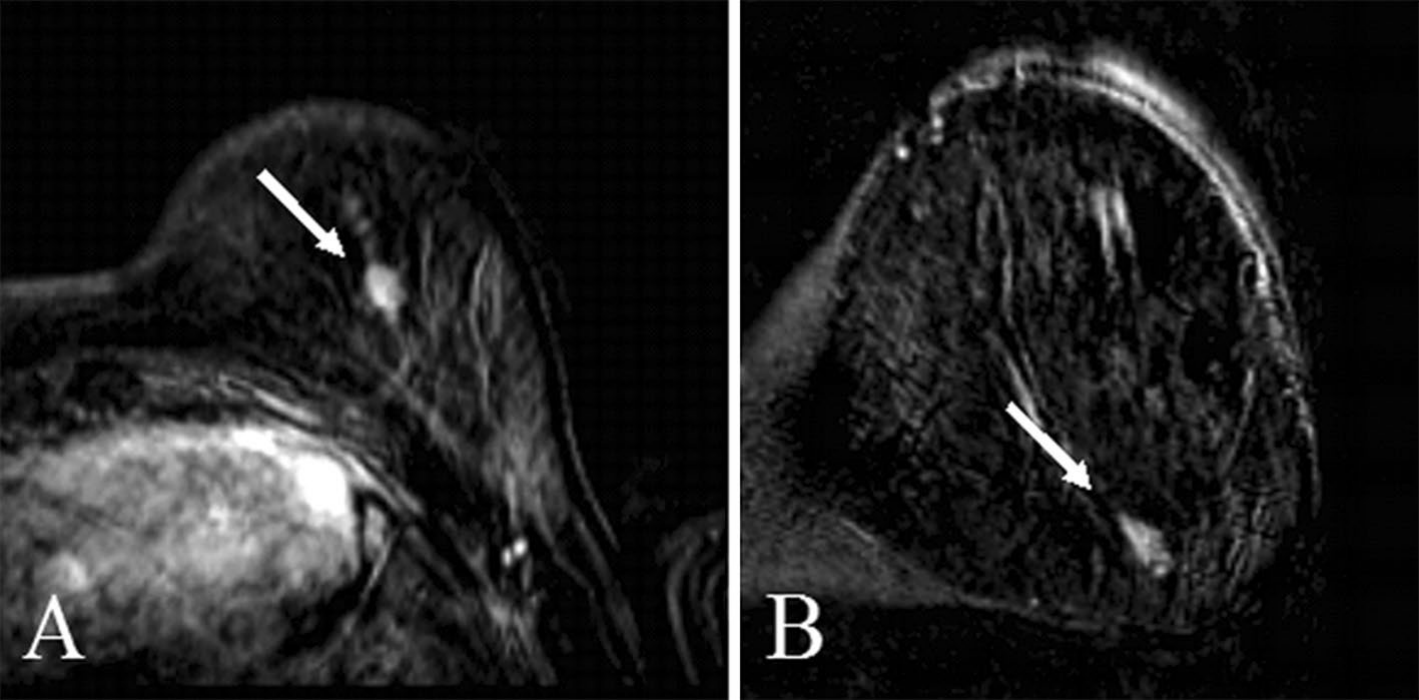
An example of an interval cancer detected on ultrasound 9 months after prior MRI. This visible lesions was scored as BI-RADS 5 in re-evaluation; a subtraction image of an invasive ductal carcinoma grade 2 (pT1cN1mi (sn)) in transversal plane and b in coronal plane
